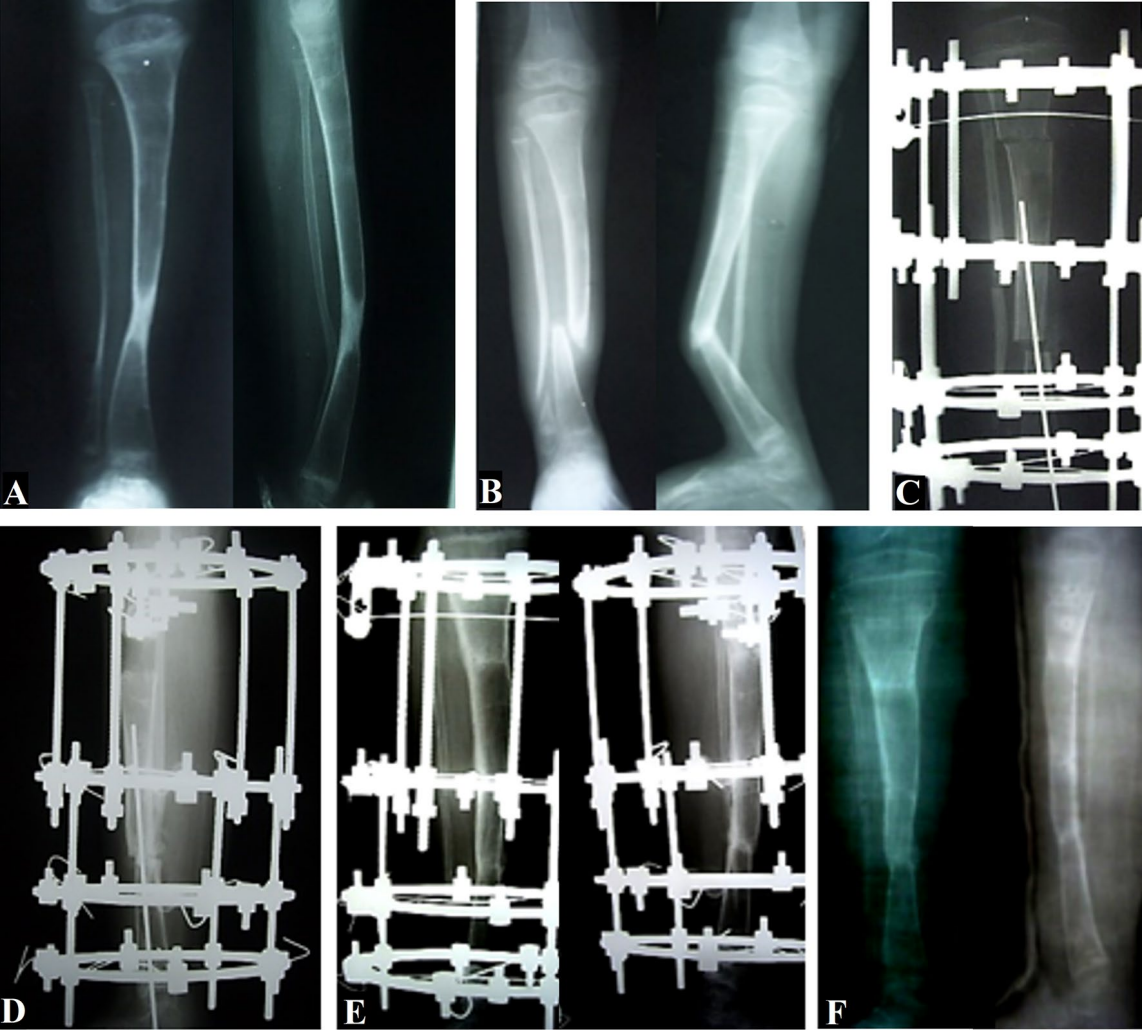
A case of a 4.5-year-old male who had undergone a previous excision of CPT when he was 3 years old. A AP and lateral X-rays showing recurrence of sclerosis and obliteration of medulla, and an impending fracture was noted. B AP and lateral X-rays showing refracture of the tibia and dysplastic fibula. C Immediate postoperative AP X-ray showing complete CPT excision, proximal corticotomy, intramedullary alignment using transcalcaneal wire, and near-total occlusion of the pseudarthrosis site by acute intraoperative shortening. D Lateral X-ray taken 8 weeks postoperatively, showing progression of union at the docking site and distraction at the regenerate. E Pre-removal AP and lateral X-rays showing complete union at the docking site and full consolidation of the regenerate. Note that the transcalcaneal wire was removed at an earlier stage. F AP and lateral X-rays taken 1 month after the removal of the frame, which show solid union without any sign of recurrence
